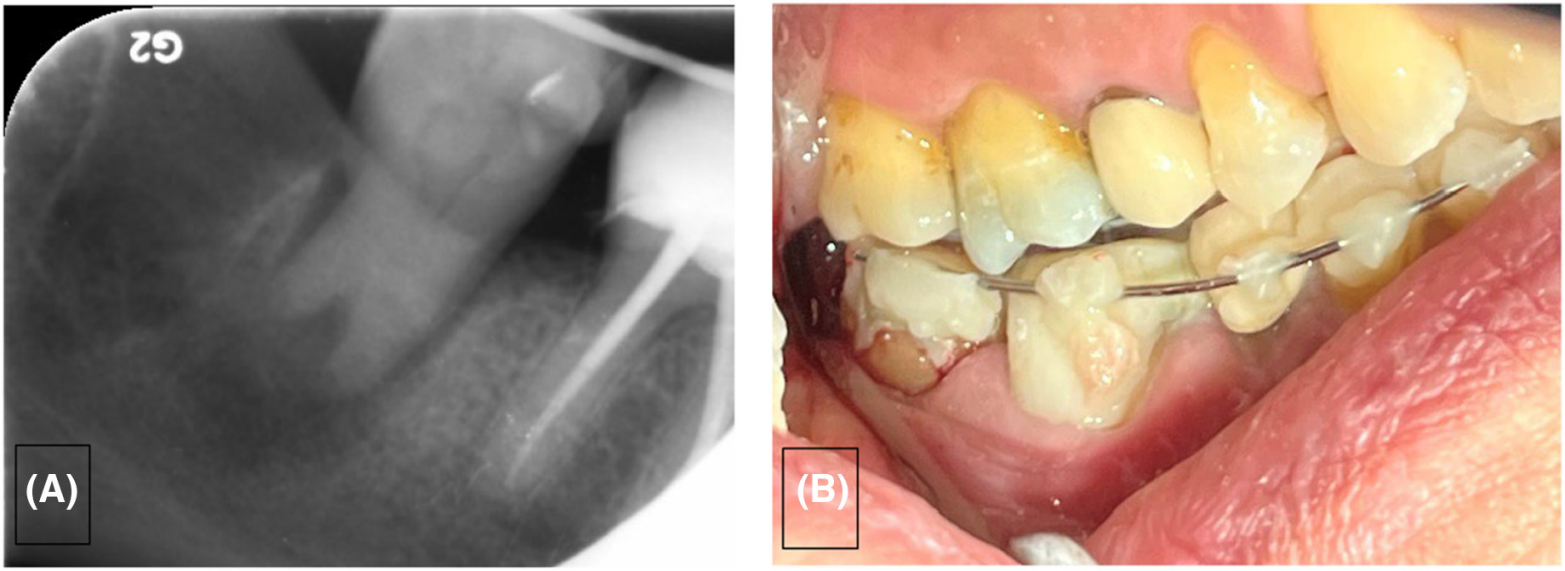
Periapical radiograph (A) and photograph image (B) taken immediately after the transplantation procedure shows the immediate post‐transplantation state of the third molar tooth.
